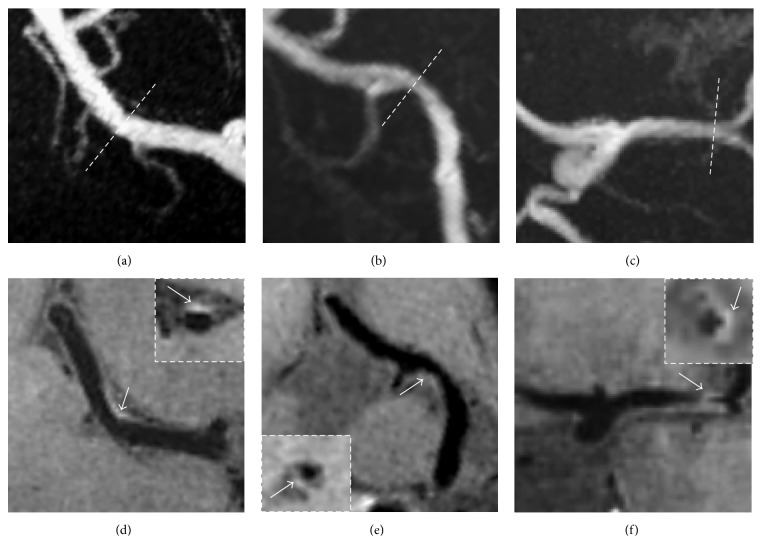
Contrast-enhanced 3D HR-MRI images from three patients. (a)–(c): MRA images show no stenosis on the relevant MCAs in these patients (*short dash line*). (d)–(f): Contrast-enhanced 3D HR-MRI images of the corresponding patients show the plaques and their positions along the MCAs (see arrow): (d) superiorly located superior; (e) inferiorly located plaque; (f) plaque involving both superior and inferior wall.
